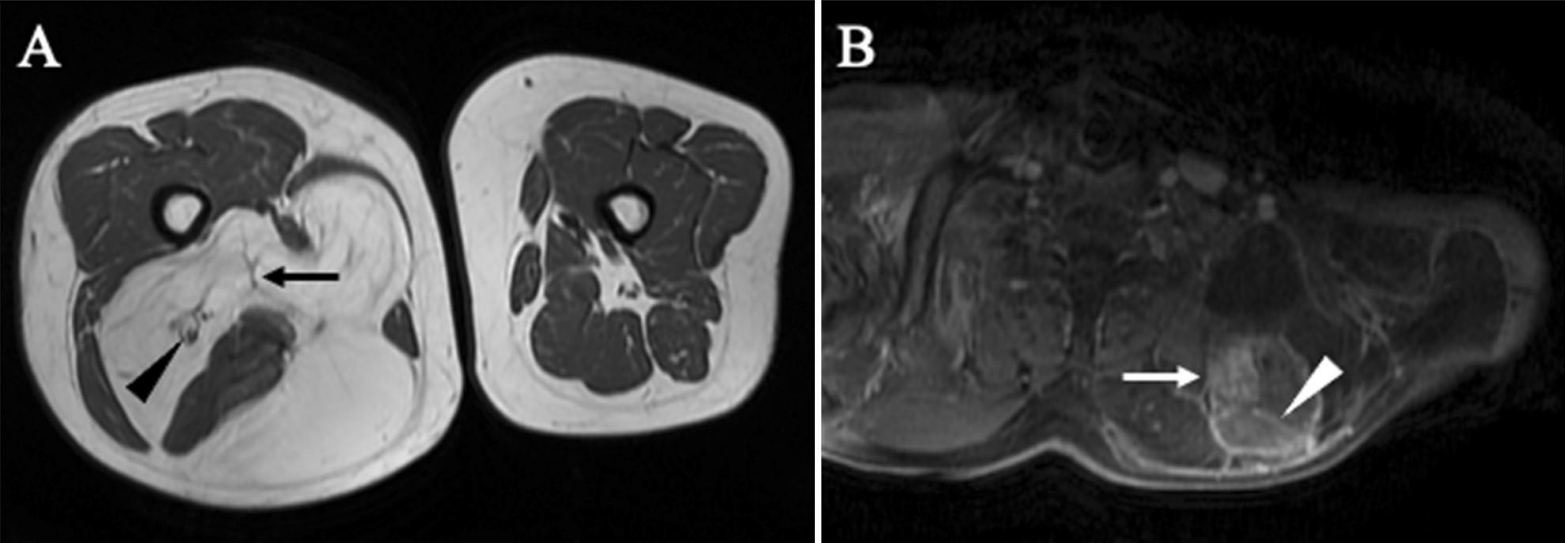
Radiological findings on MRI used in this study. (A) Thick septa (> 2 mm) (black arrow) and the sciatic nerve involved in the adipocytic tumor (black triangle) in T1-weighted image. (B). Enhancement of septa (white triangle) and nodular lesion (white arrow) in contrast-enhanced fat-suppression T2 image.
